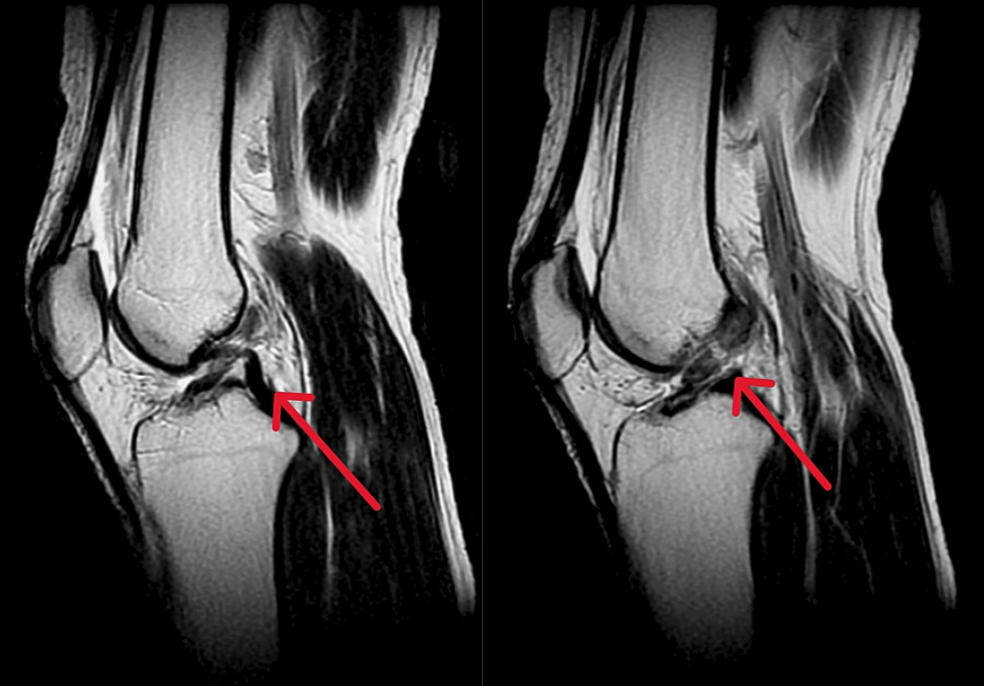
Pre-operative MRI of knee joint MRI revealing partial tear of ACL along with complex tear of medial meniscus ACL, anterior cruciate ligament
